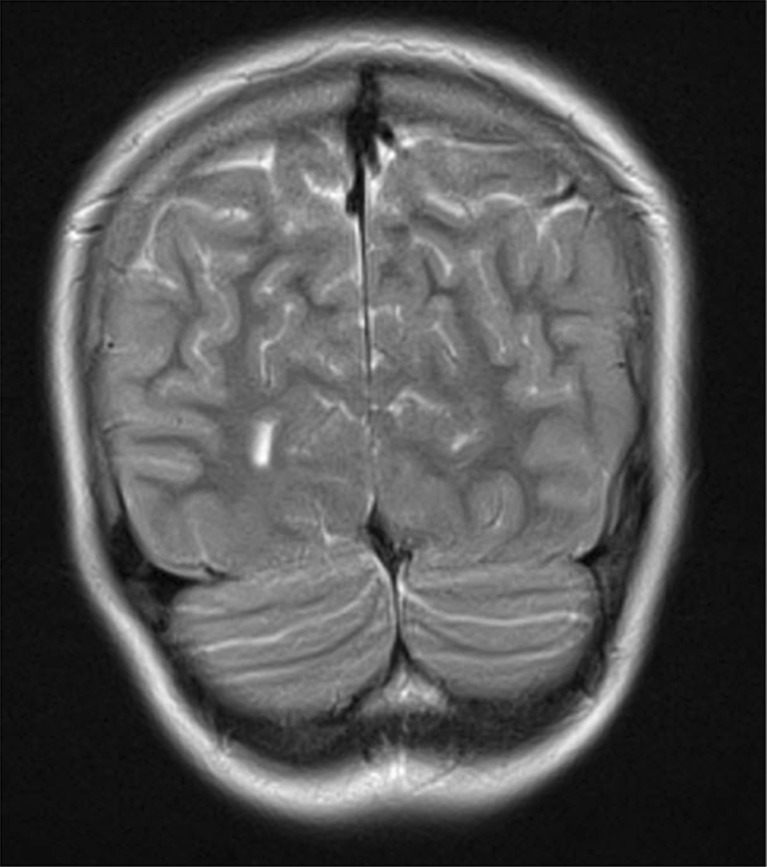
Calcifications in basal ganglia on computed tomography
